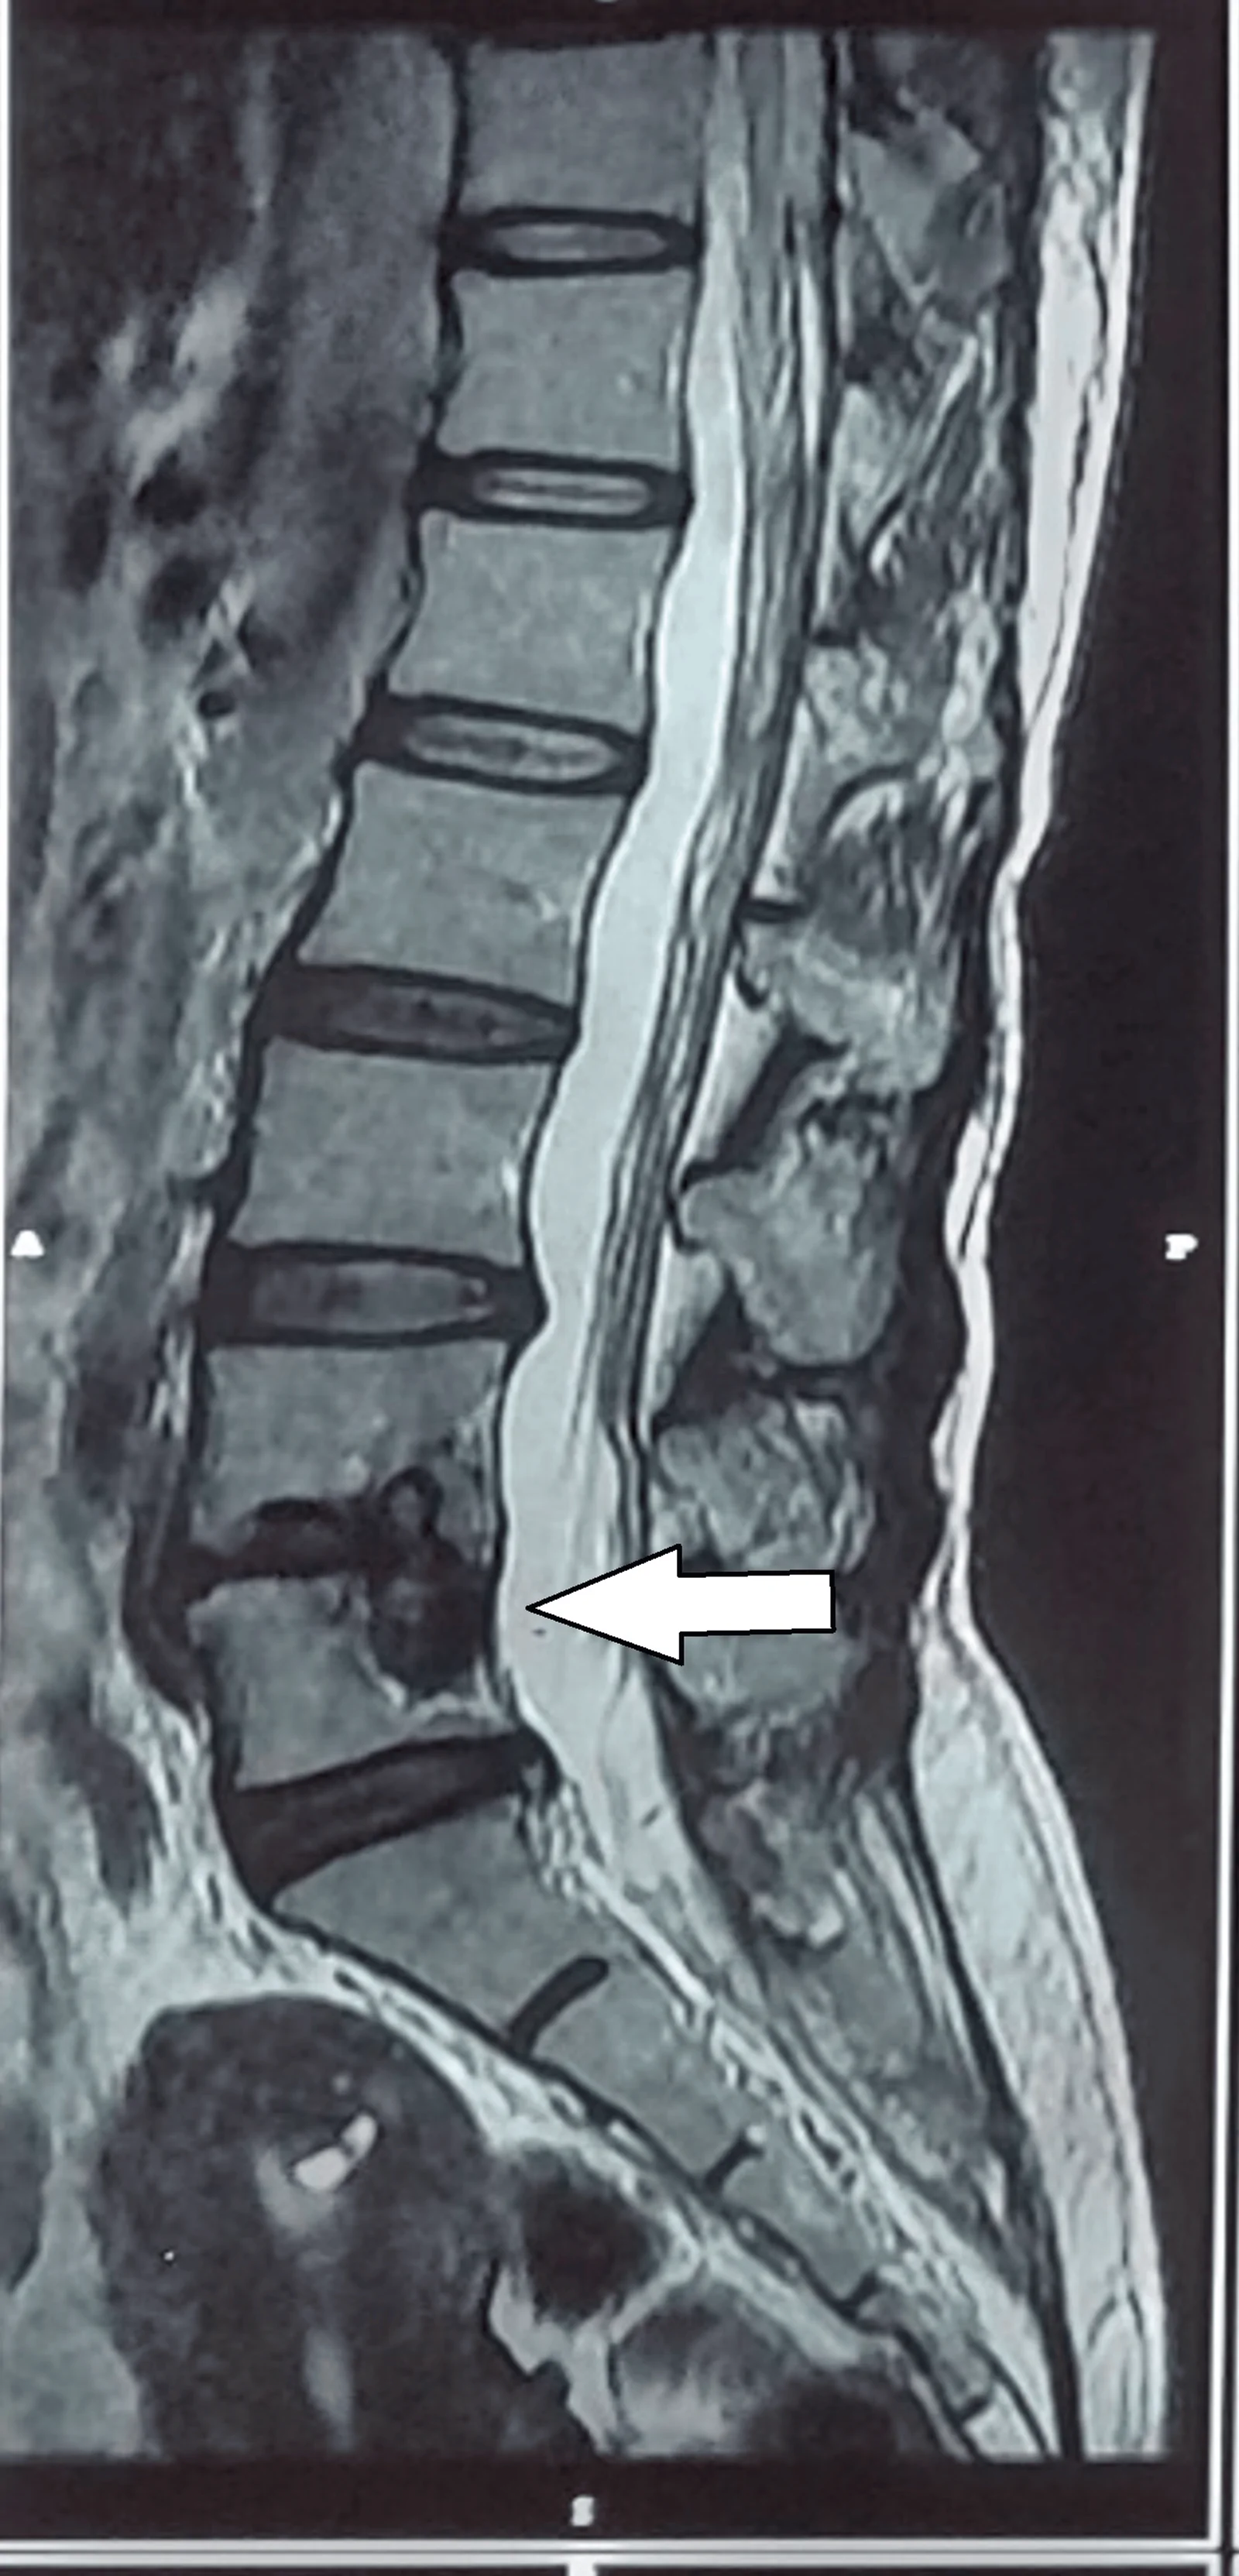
MRI LS spine (sagittal view) showing multiple lytic lesions in L4-L5 vertebrae (solid white arrow) MRI: magnetic resonance imaging; LS: lumbosacral
